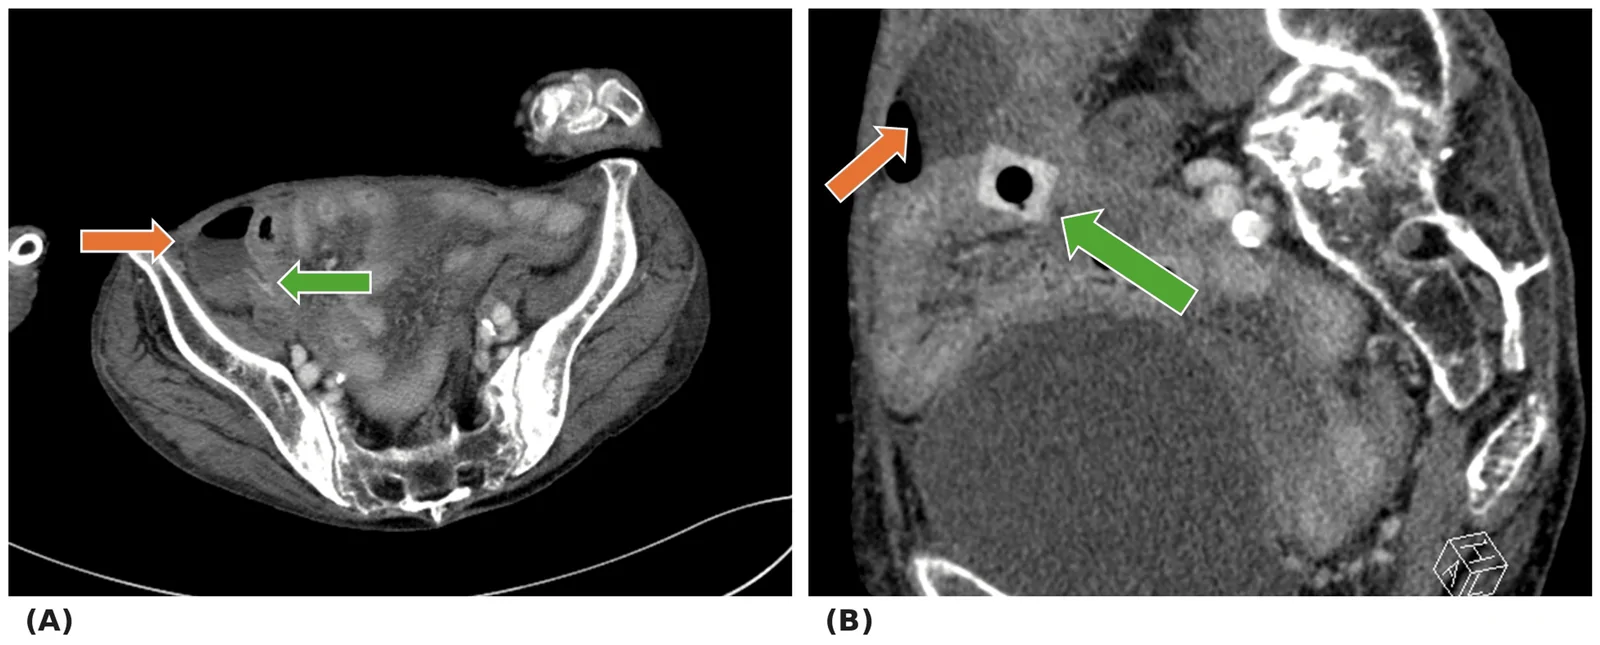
Contrast-enhanced abdominal computed tomography findings (A) Axial image. The orange arrow indicates the abscess cavity between the abdominal wall and the bowel in the right lower quadrant. The green arrow indicates the retained PTP within the ileum approximately 10 cm proximal to the ileocecal valve, identifiable as a high-density tablet surrounded by a low-density halo corresponding to the air pocket within the PTP dome. (B) Reconstructed image. The orange arrow again demonstrates the abscess cavity. The green arrow identifies the PTP, where its morphology is more clearly delineated on this projection. PTP: press-through package
